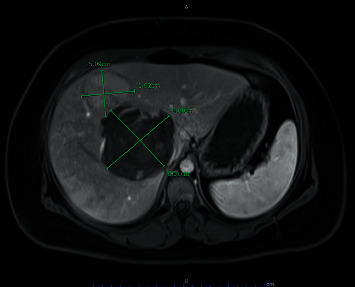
MRI of the liver with contrast showing a sizable hemorrhagic HA measuring 9.0 × 8.5 cm and adjacent nonhemorrhagic HA 5.9 × 5.1 cm (segment 4) and several HAs smaller than 5 cm in diameter.
